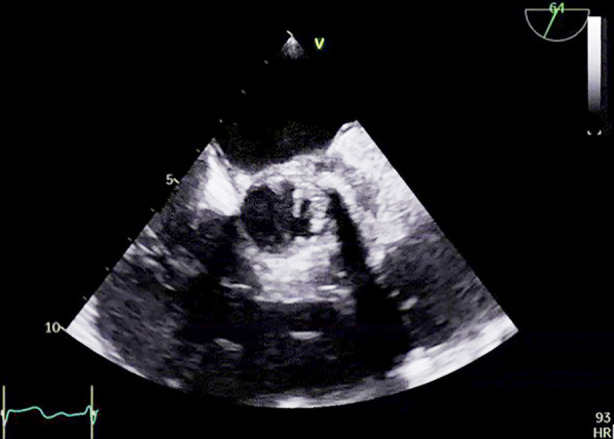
Transesophageal echocardiogram, showing aortic graft vegetations and peri-aortic graft thickening.
